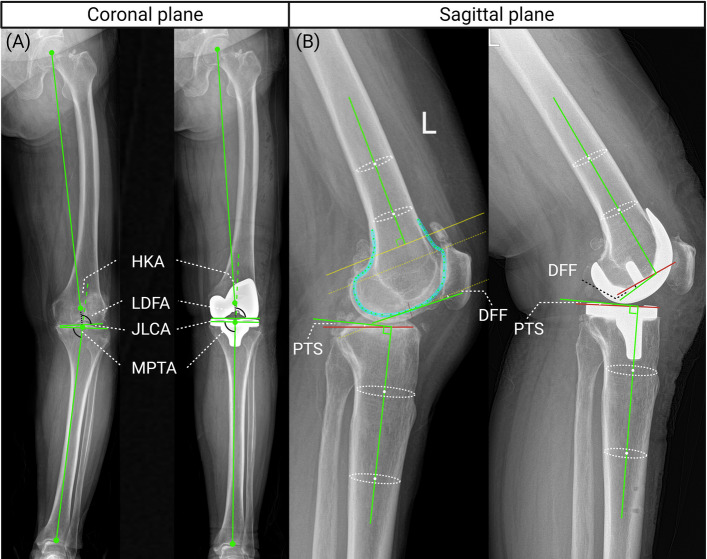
Radiographic measurement of coronal and sagittal plane parameters. A Coronal plane parameters measured on standing full-length lower extremity radiographs, including hip-knee-ankle angle (HKA), lateral distal femoral angle (LDFA), medial proximal tibial angle (MPTA), and joint line congruency angle (JLCA). B Sagittal plane parameters measured on standard lateral knee radiographs with adequate femoral condyle overlap, including posterior tibial slope (PTS) and distal femoral flexion (DFF)
